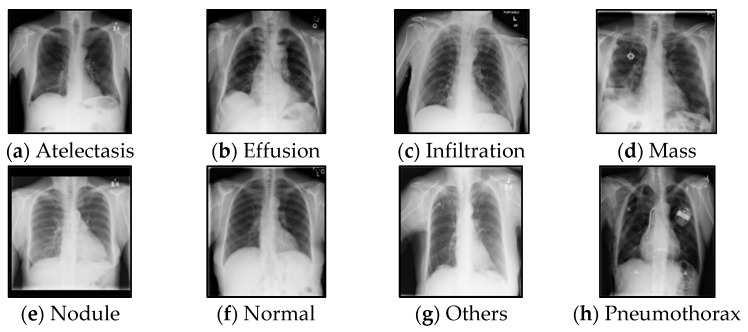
The chest X-ray sample images.
